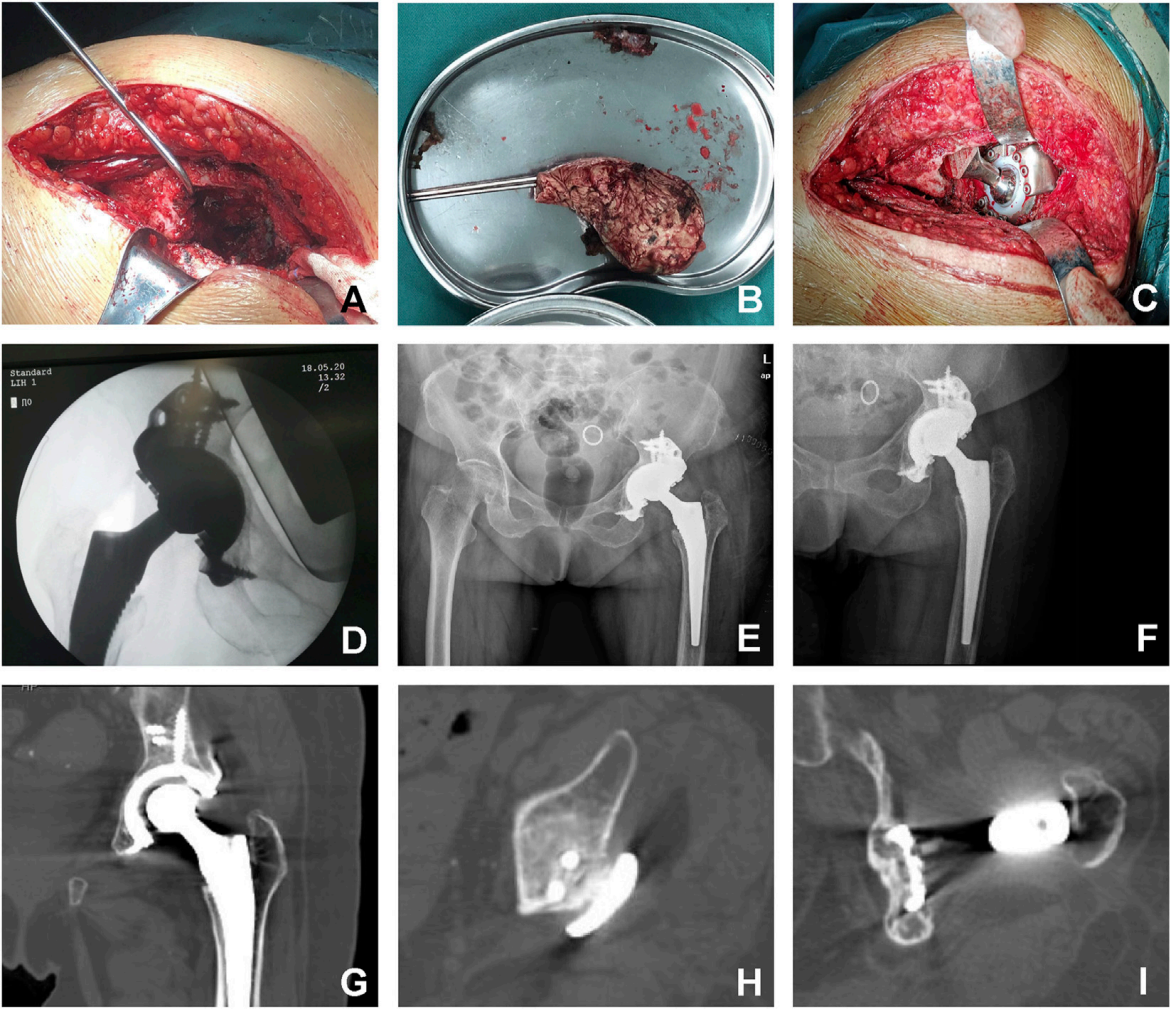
Surgical Procedure and Radiographic Follow-up. (A) Removal of cement spacer and exposure of acetabular bone defect morphology. (B) Extracted cement spacer. (C) Implantation of the 3D-printed triflange acetabular component. (D) Intraoperative fluoroscopy confirming optimal prosthesis positioning. (E) Postoperative day 3 anteroposterior radiograph. (F) 4-year postoperative anteroposterior radiograph. (G–I) Computed tomography (CT) scans at 4-year follow-up demonstrating osseointegration of the acetabular cup and flanges.
